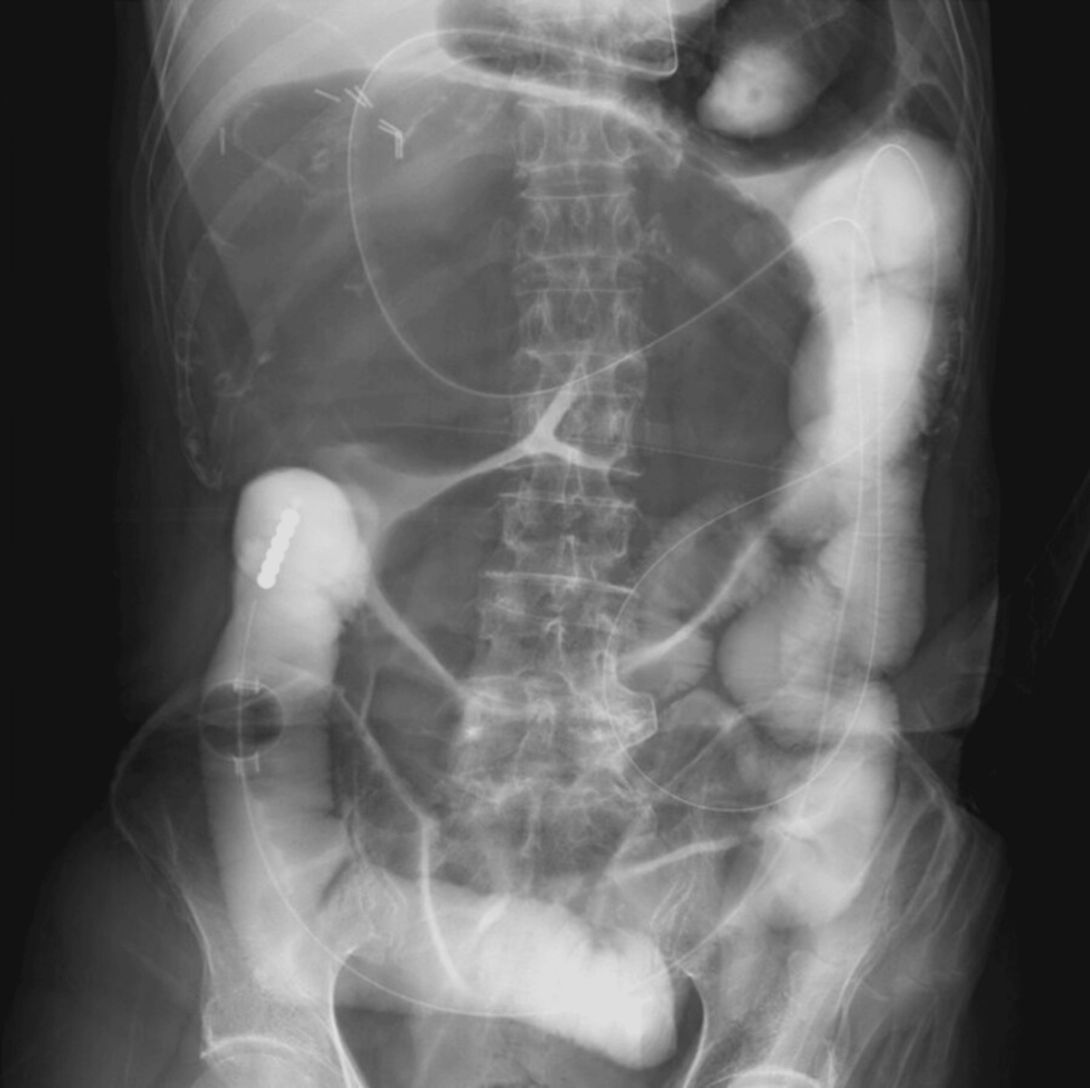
Transnasal ileus tube insertion proximal to the dilated colon Abdominal radiograph showing transnasal ileus tube positioned in the small intestine proximal to the dilated cecum.
